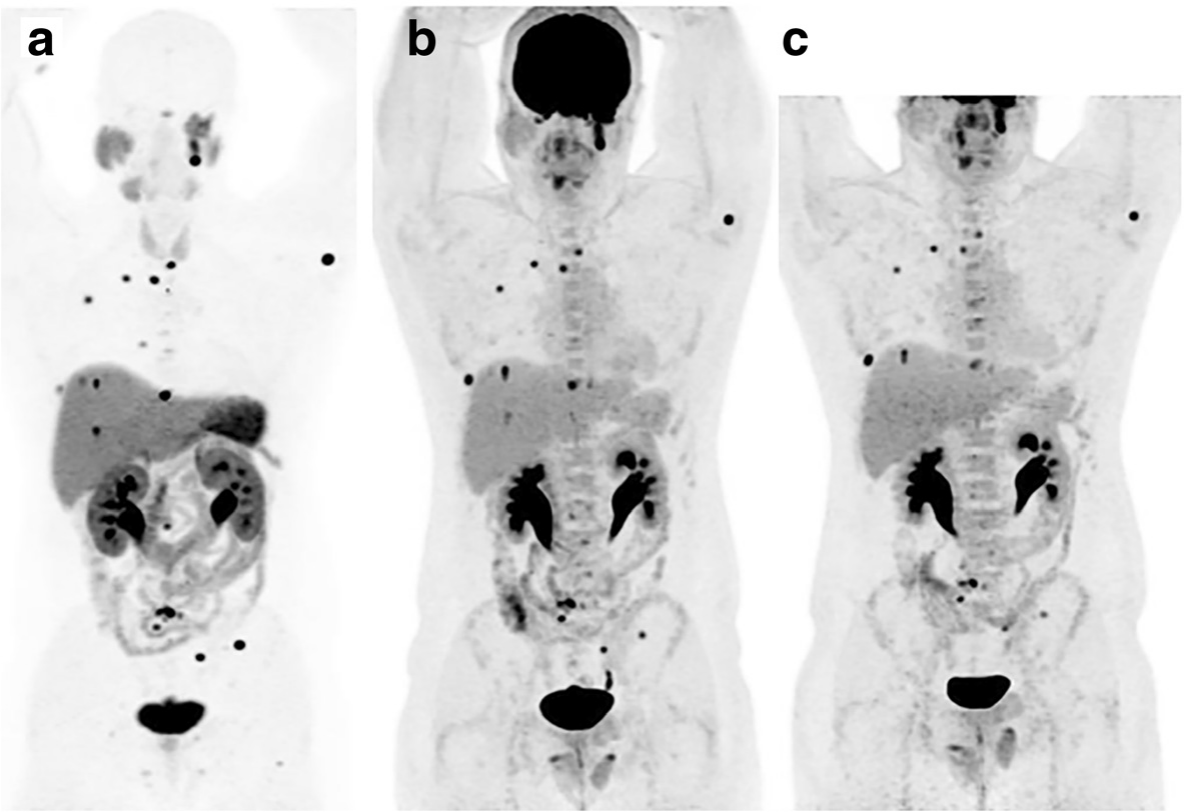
A 20 year old male with *SDHB* germline mutation and metastatic PCC. Baseline Ga-68 DOTATATE (a) and F-18 FDG (b) demonstrated metastatic disease with a concordant pattern of uptake. The patient was treated with 3 cycles of peptide receptor radionuclide therapy for uncontrolled hypertension. Follow-up DOTATATE and FDG imaging over a 2 year period demonstrated stable findings (c: FDG at 2 years). The high FDG-uptake was not predictive of an aggressive phenotype and reflects changes secondary to *SDHB* mutation and consequent disordered oxidative phosphorylation
